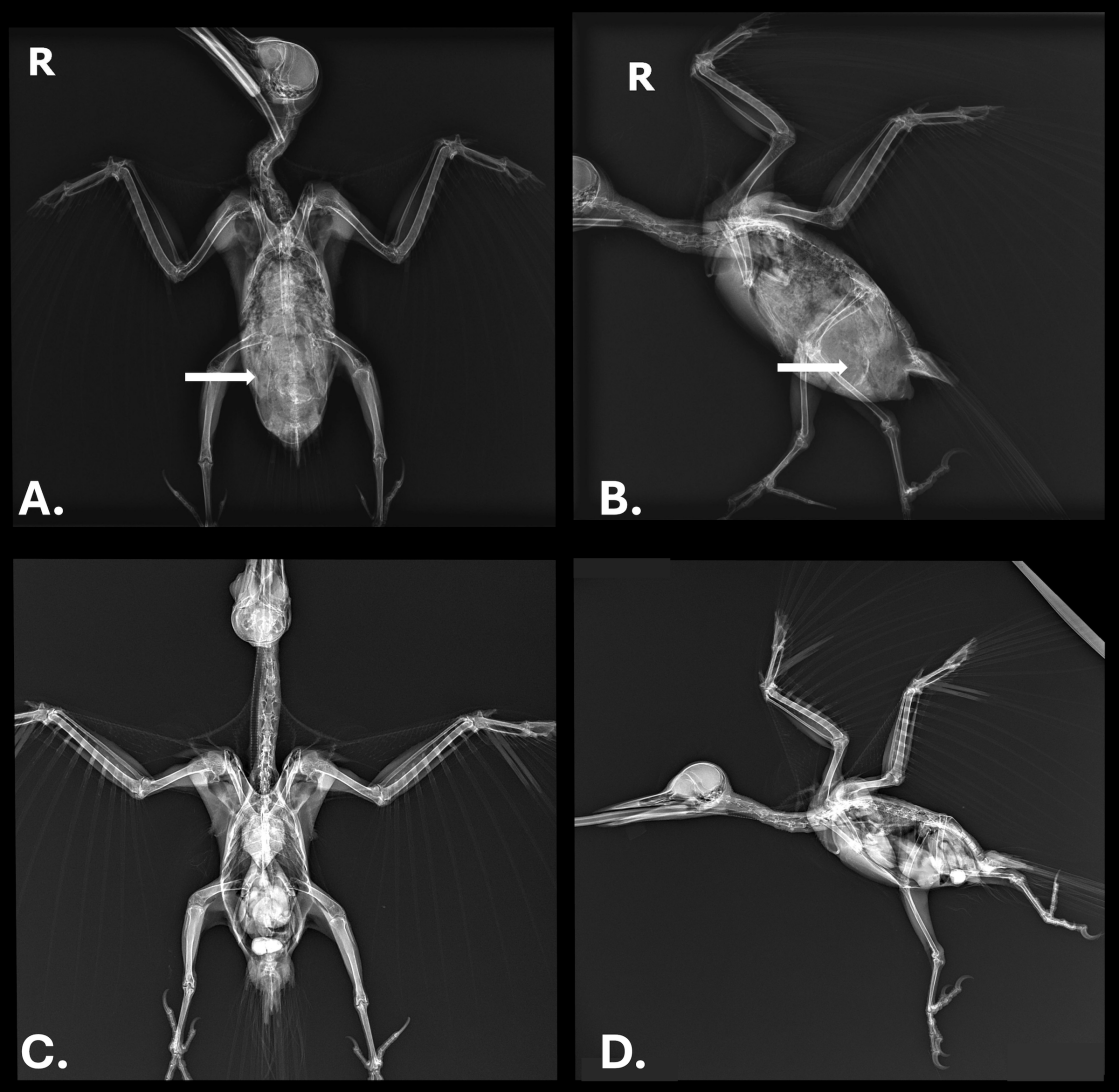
(A) Ventrodorsal view and (B) lateral view radiographs of a pileated woodpecker (*Dryocopus pileatus*) with high burden of a *Diplotriaena* sp. White arrows represent where air sac worms are present; only one side in A is marked for simplicity. (C, D) Radiographs of an uninfected pileated woodpecker showing the same region where air sacs are clear instead of opaque.
